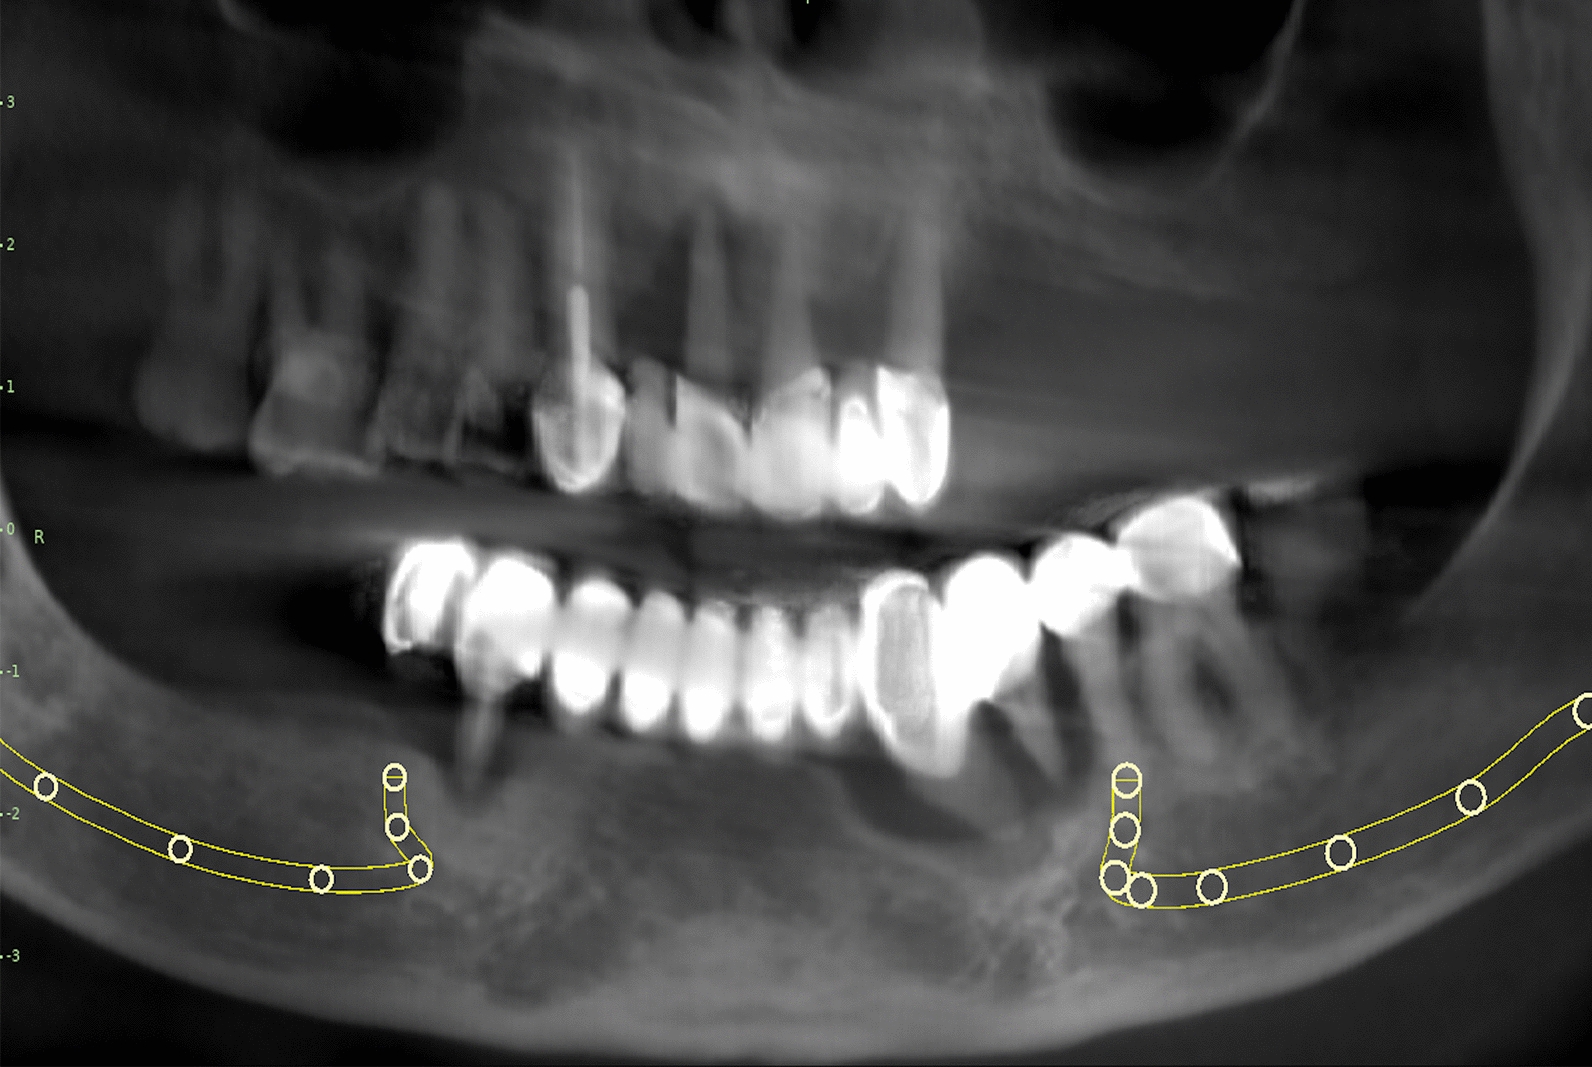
Panoramic X-ray showing bone loss around the natural teeth for a patient having long-span fixed prosthesis
